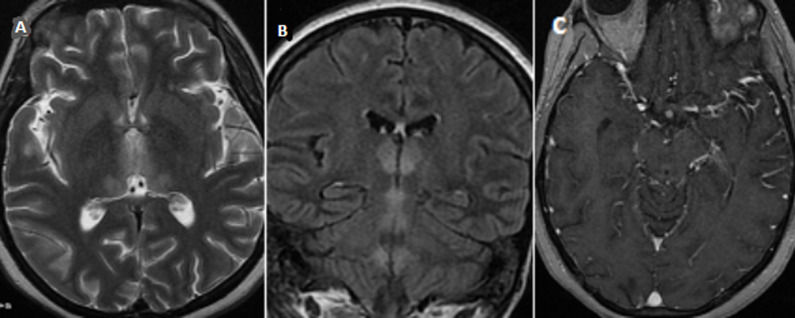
IRM cérébrale montrant un hypersignal T2 (A) et FLAIR (B) bilatéral symétriques des 2 thalamis, des régions périventriculaires, des parois du 3^e^ ventricule et des tubercules mamillaires avec discrète prise de contraste de la région périaqueducale
